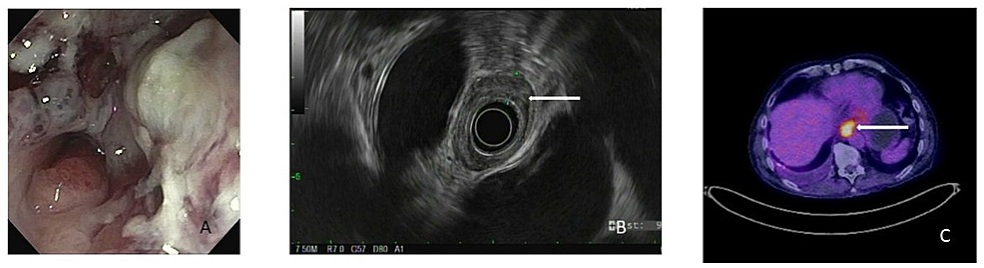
Representative images of esophageal cancer on endoscopy (A), EUS (B) and PET scan (C). EUS: endoscopic ultrasound; PET: positron-emission tomography.
